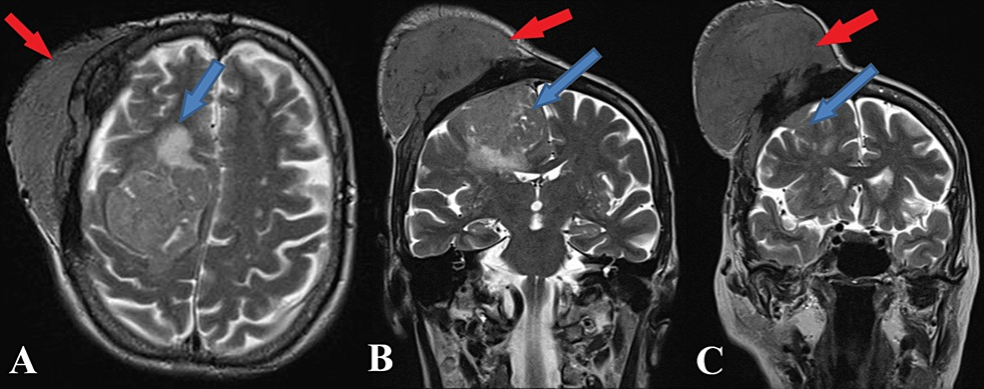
Preoperative MRI T2 FLAIR sequence Axial section (A). Coronal section (B,C). The arrows point to a giant intracranial (blue arrows) and extracranial (red arrows) meningioma with significant osteolysis of the skull after extensive extracranial invasion.
